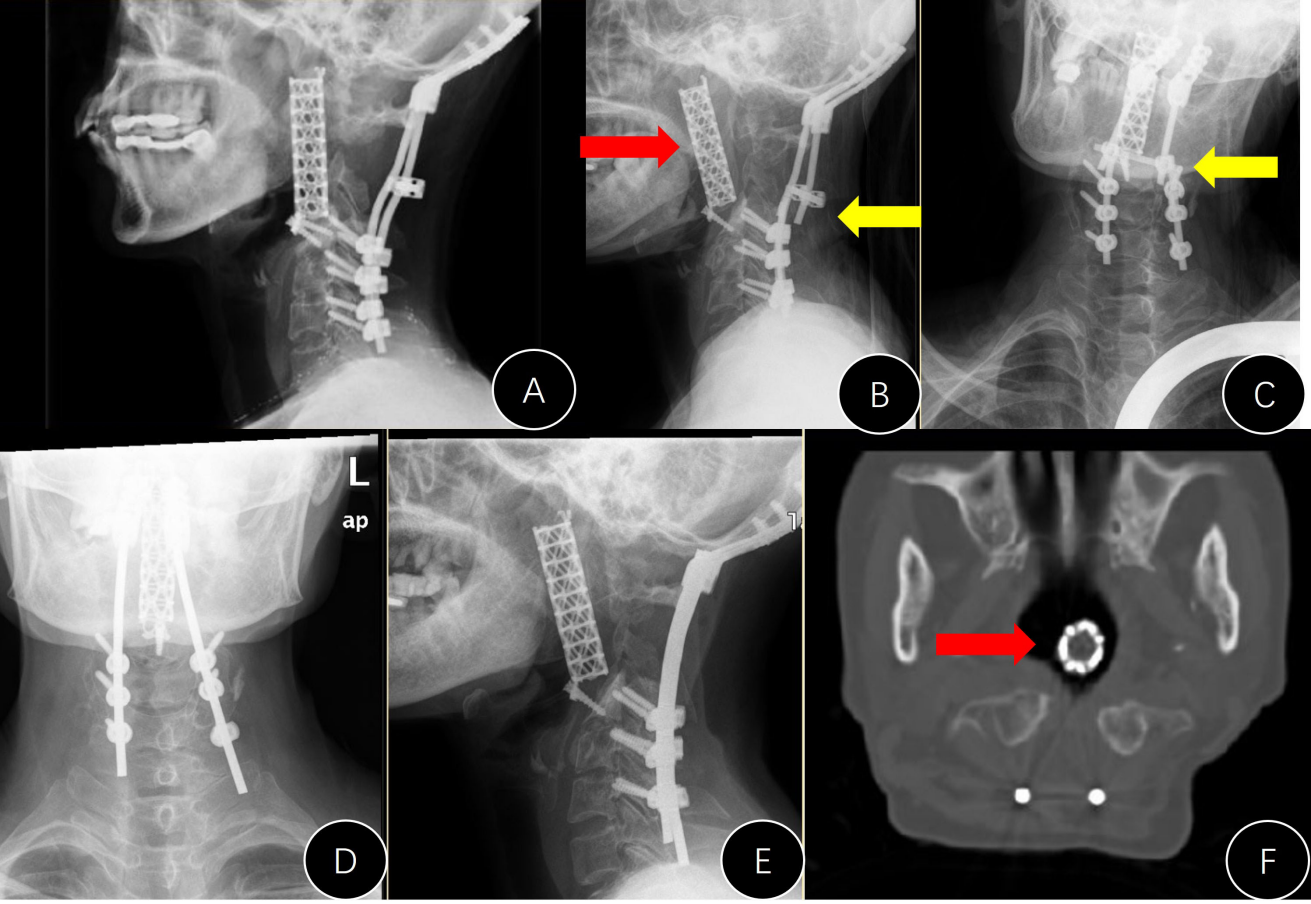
Presentation of case #A6. (A) Postoperative lateral x-ray; (B, C) x-rays 7 years later (anterior movement of titanium mesh and the breaking of both rods); (D, E) revision surgery to replace the rods; (F) mesh shifted into the pharyngeal cavity.
